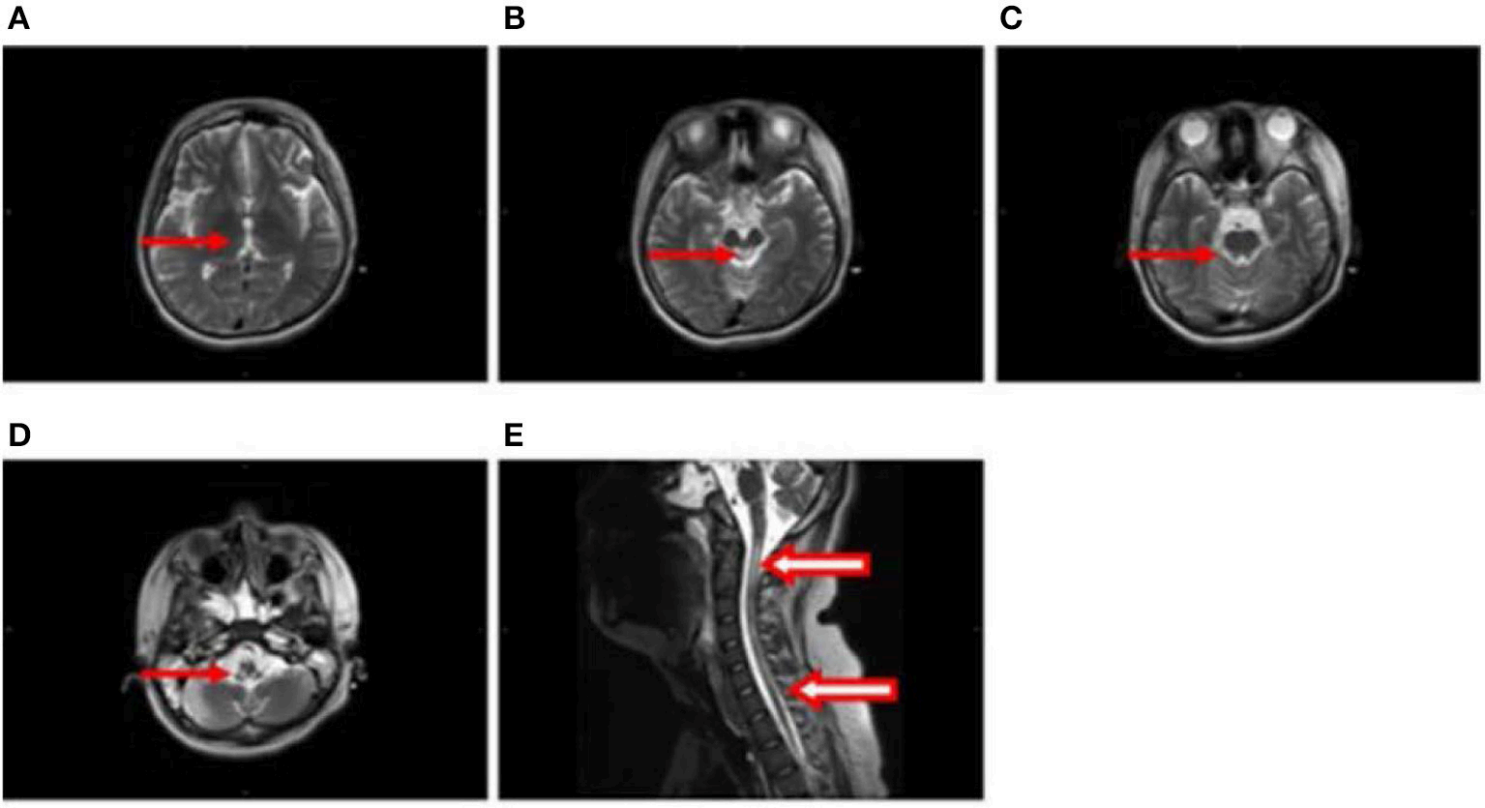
(A–E) Axial and sagittal T2-weighted MRI at 7 months following hospitalization revealed hyperintensities in the bilateral thalamus (arrow in A), tegmental area of the midbrain (arrow in B), pons (arrow in C), medulla (arrow in D), and the cervical and thoracic spinal cord (new lesions, hollow arrow in E). The previously identified lesions had decreased in size because of atrophy.
